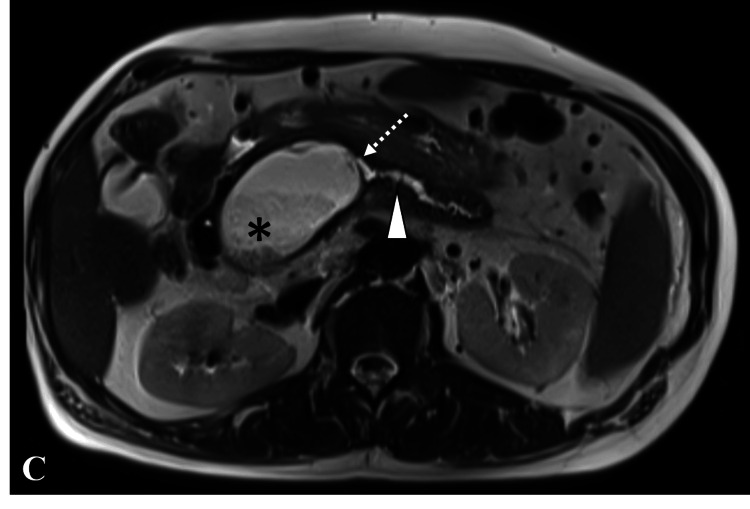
Axial T2-weighted MRI confirming a pancreatico-portal fistula with intraluminal portal vein debris. Dashed white arrow indicates the pancreatico-portal fistulous communication; black asterisk indicates dependent debris within the portal vein; white arrowhead indicates the dilated main pancreatic duct.
